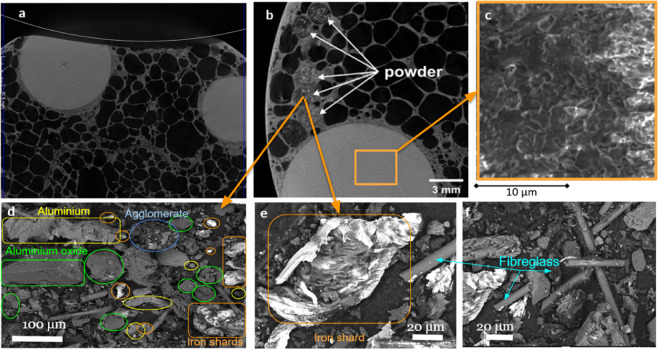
(a,b) Computed tomography scans of the cross sectional area of the cylindrical sample; (c) SEM image of ceramic spheres with the surface morphology consistent with low densification ceramic structure; (d–f) SEM images of the particulate, which included aluminum oxide, aluminum, iron shards, and the fractional content of the blade composition such as fiberglass fragments, titanium dioxide, calcium oxide carbonate, magnesium oxide, graphite as well as fluorides.
